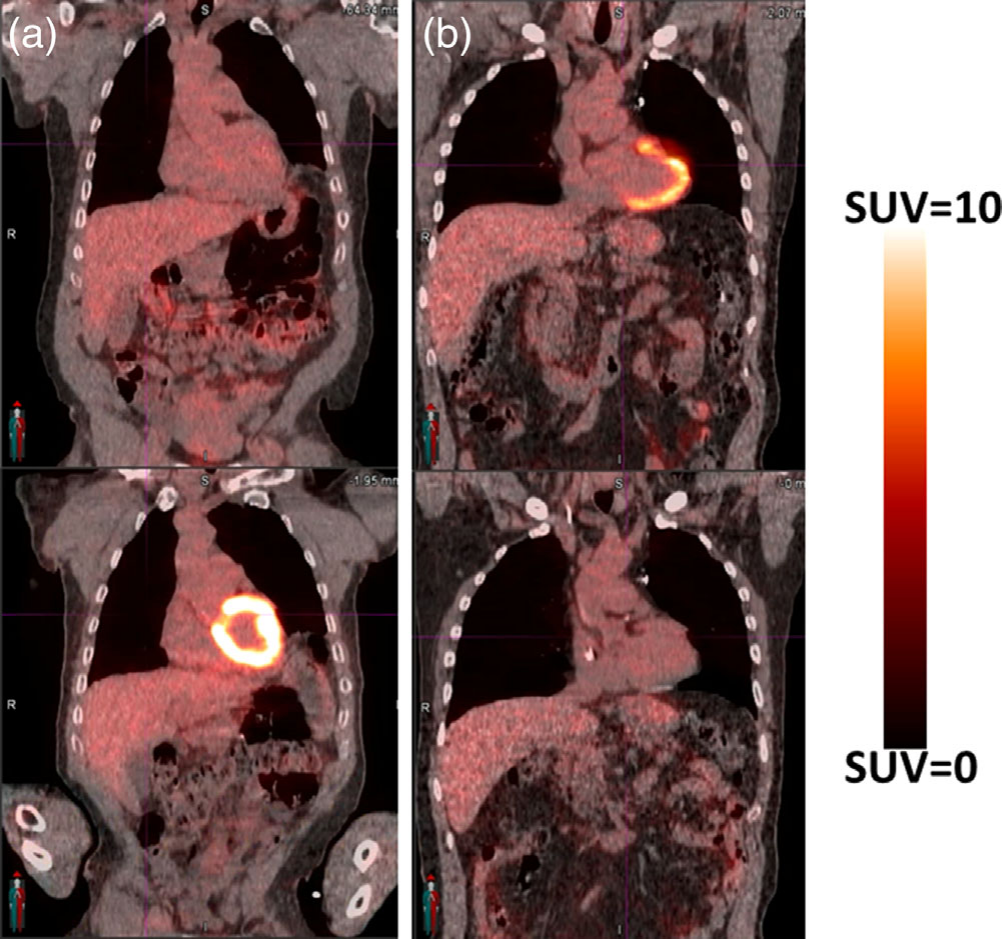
Patient examples of a patient with (a) increased post‐treatment cardiac SUV compared to pre‐treatment cardiac SUV and (b) decreased post‐treatment cardiac SUV when compared to pre‐treatment. SUV, standard uptake value
